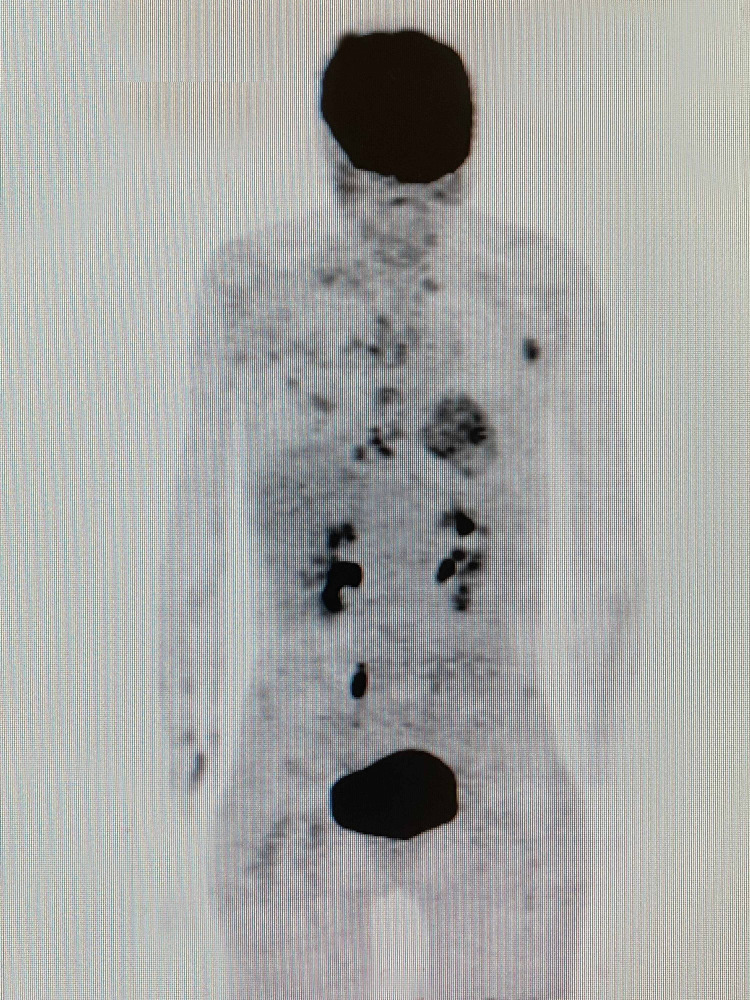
Whole body (18F)-FDG-PET-CT scan done after four cycles of MSCT, KD, HT, and HBOT treatment (January 16, 2019) showing a partial response to treatment of the metastatic liver lesions and left axillary LAP and complete response to treatment at all other lesions (18F)-FDG-PET/CT: 18F-fluorodeoxyglucose positron emission tomography/computed tomography; HBOT: hyperbaric oxygen therapy; HT: hyperthermia; KD: ketogenic diet; MSCT: metabolically supported chemotherapy
